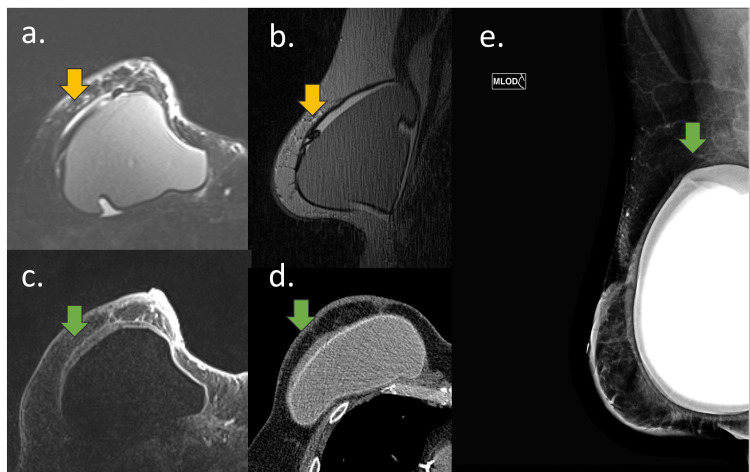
Early complications of acellular dermal matrix (ADM) A 55-year-old woman with an adenomastectomy reconstructed with a breast implant and ADM for one year. (a) Axial STIR-weighted sequence, (b) sagittal DP weighted sequence, (c) axial post-contrast sequence, (d) breast tomography, and (e) mammography oblique view MRI: (a, b, and c) Foldable intracapsular hyposignal tissue (yellow arrow) associated with intracapsular collection in green arrow. CT: (d) Intracapsular collection with heterogeneous material in green arrow and (e) intracapsular collection (green arrow) in mammography. The implant is rotated.
